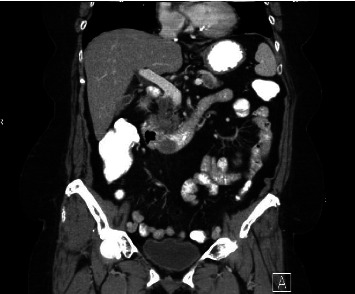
CT abdomen showing 2.5 cm duodenal wall mass.
